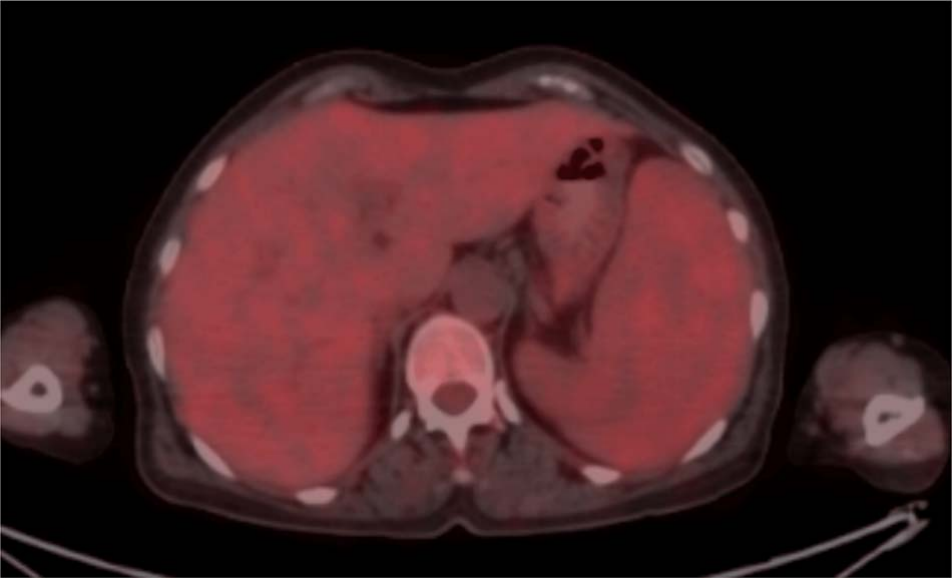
FDG-PET/CT revealed a diffusely mild hypermetabolic appearance in the splenic parenchyma, comparable to the liver.
